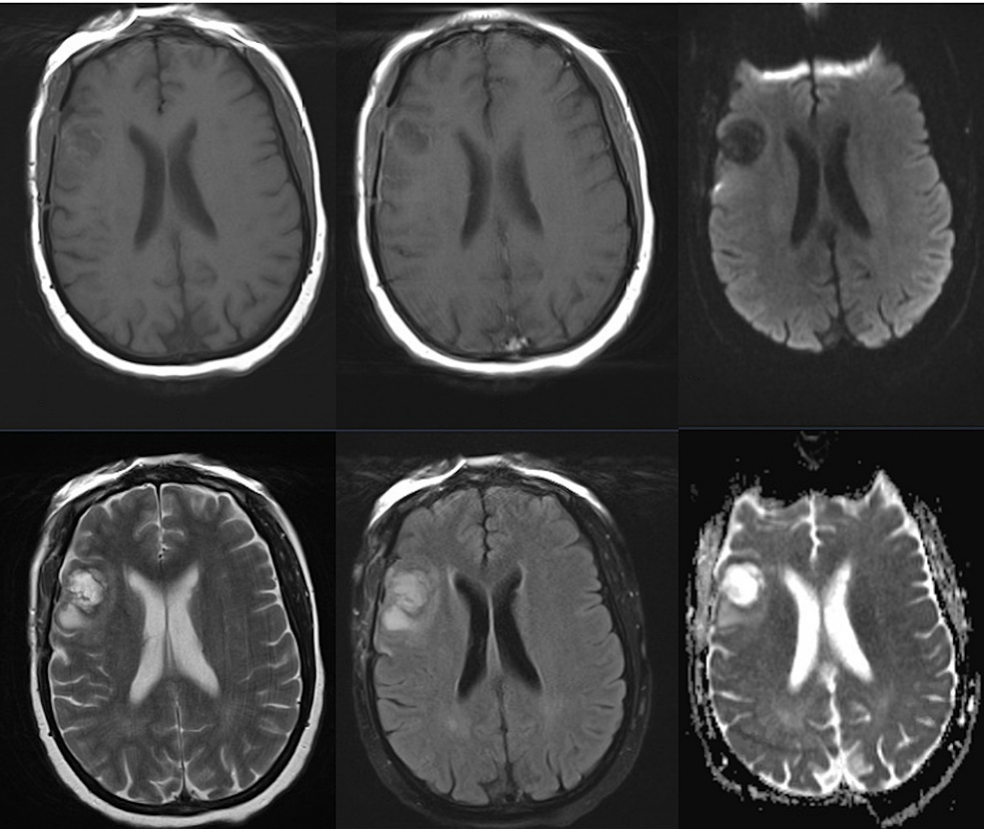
Two months postoperative MRI scan. Two months postoperative loss of enhancement of the residual abscess and minimal edema. First row: Two months postoperative T1-weighted, T1 + contrast, diffusion imaging. Second row: Two months postoperative T2-weighted, FLAIR, and ADC ADC: apparent diffusion coefficient; FLAIR: fluid-attenuated inversion recovery; MRI: magnetic resonance imaging
